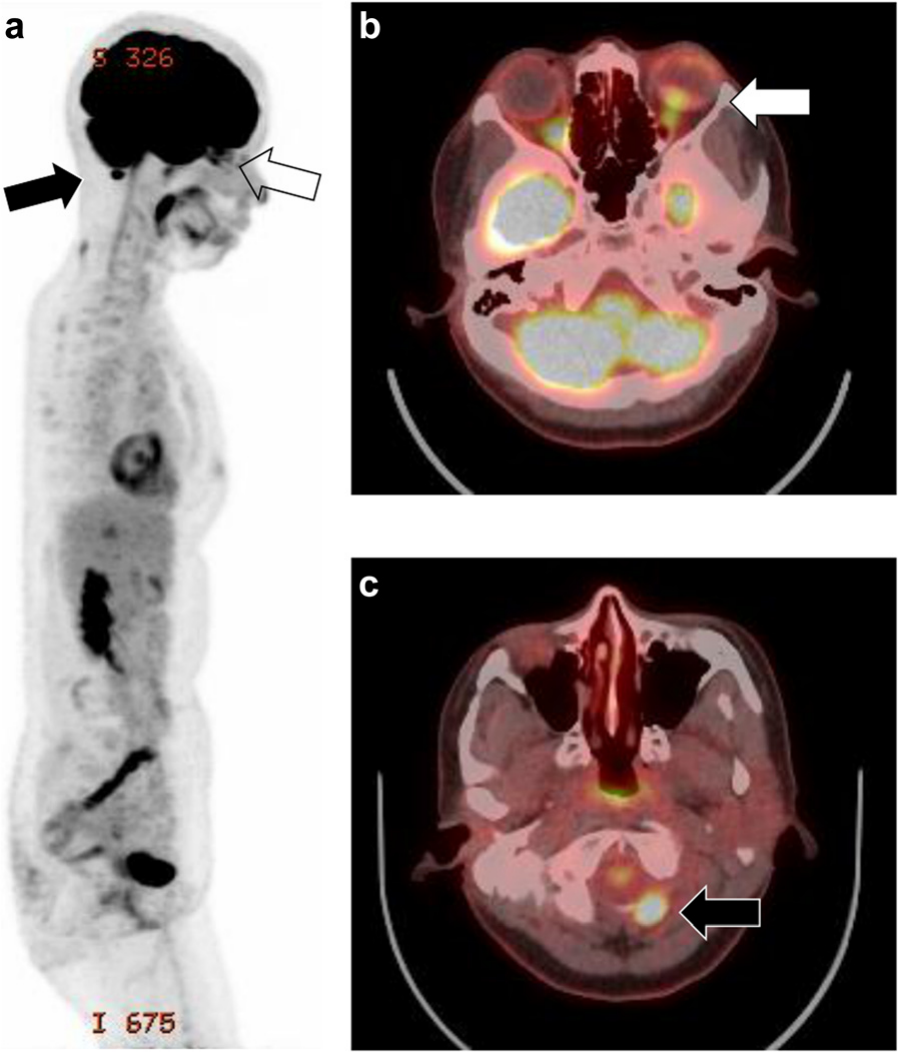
FDG PET/CT sagittal image (a) revealed two focal regions of increased uptake in the eye (*white arrow*) and posterior neck (*black arrow*). Axial image (b) showed increased FDG uptake in the eye (*white arrow*) and the choroid plexus of the left eye (SUVmax = 3.8 g/ml). Axial image (c) of the first cervical (C1) spine showed increased uptake (*black arrow*) adjacent to the C2 lamina (SUVmax = 9.9 g/ml)
